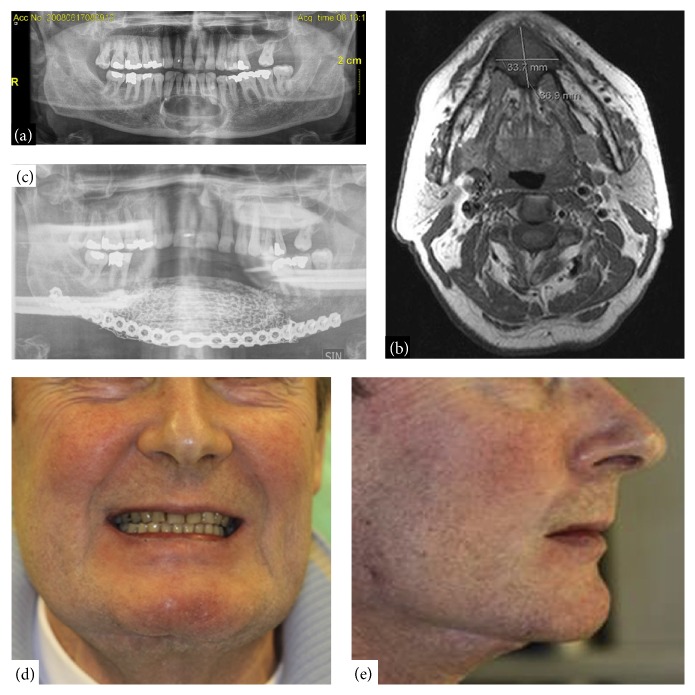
Tissue-engineered ASCs could be utilized to treat a large anterior mandibular defect. (a) X-ray image shows a nearly 10 cm recurrence of an ameloblastoma in the parasymphyseal area of the anterior mandible. (b) T1-weighted magnetic resonance image shows a large tumor (ameloblastoma) in the anterior mandible. (c) X-ray image shows the six implant fixtures inserted into the grafted site. (d) The reconstructed chin taken from the front. (e) The reconstructed chin taken from the lateral side (adopted from [65]).
